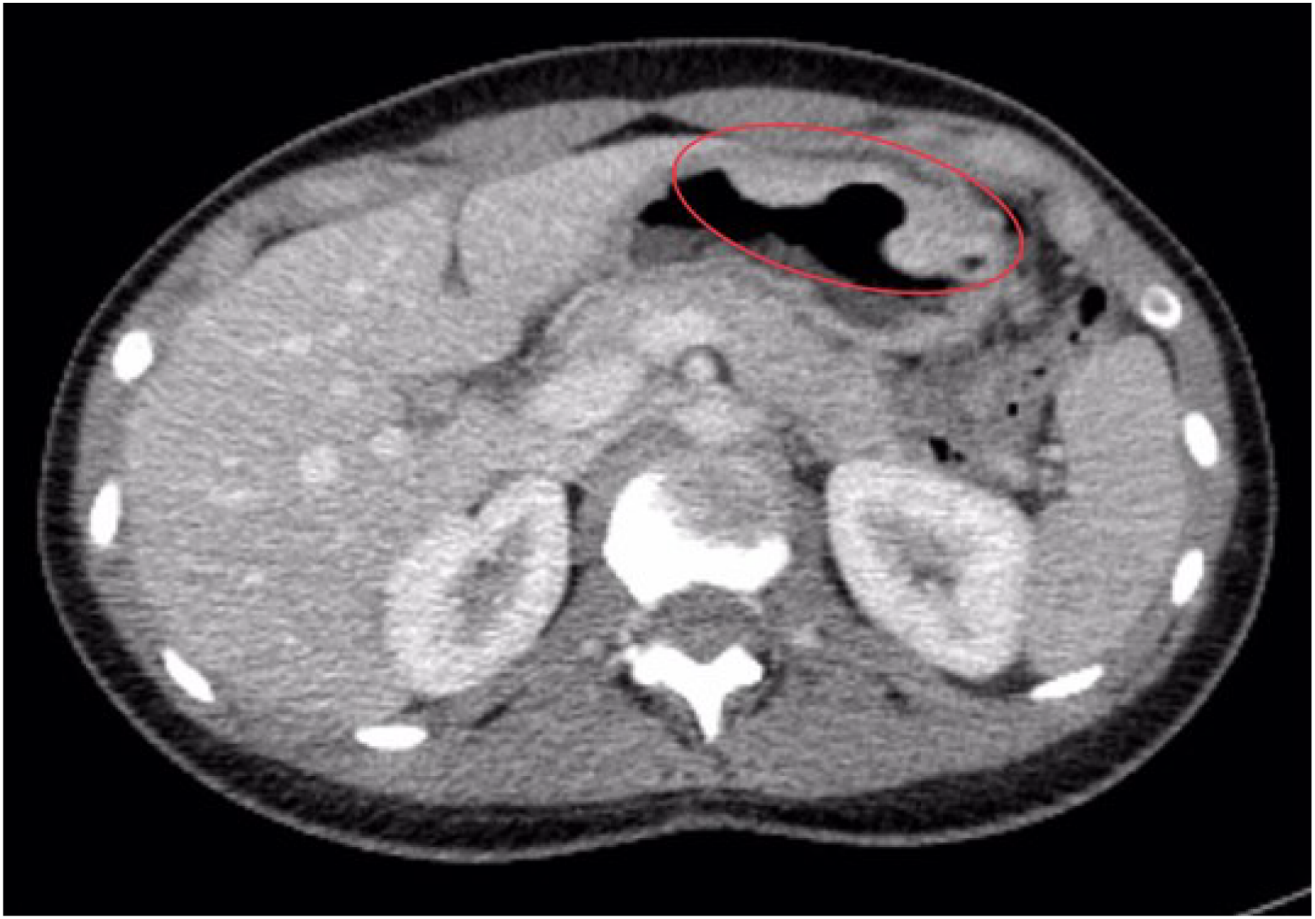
Axial contrast-enhanced CT scan (portal venous phase) showing focal nodular thickening along the gastric greater curvature. The lesion measures approximately 11 mm in thickness, with a focally exophytic contour and mild iodophilia. Its bosselated, endoluminally protruding appearance raised suspicion for a primary gastric malignancy.
